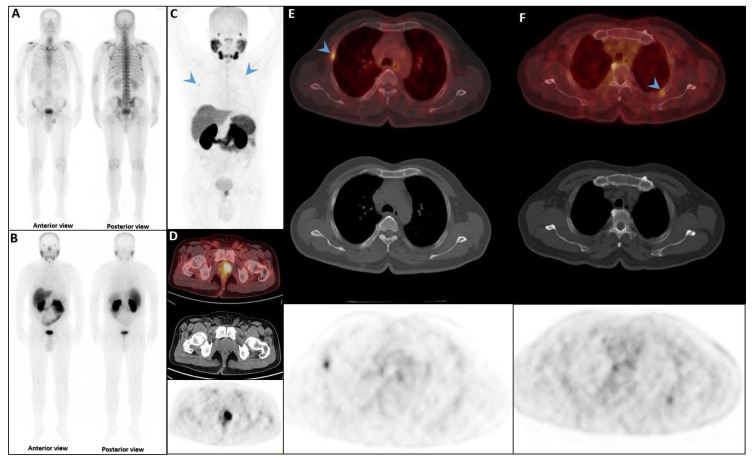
Complete discordant findings between PSMA and bone scan. 61-year-old male referred for staging that transrectal ultrasound-guided prostate biopsy showed GS 4 + 3 (12 of 12 cores involved), perineural invasion (+), and serum PSA level = 17 ng/mL. A whole-body bone scan revealed multiple increased tracer uptake in bilateral rib (A). 99mTc-PSMA SPECT/CT revealed no abnormal tracer uptake (B). Considering discordant findings in the 99mTc-PSMA and bone scan, the referring physician decided to request a 68Ga-PSMA PET/CT. The scan showed (C) multiple foci of increased activity in the left side of the prostate gland (D) as well as two foci of increased activity in bilateral ribs with no density changes on the corresponding CT images (SUVmax up to 3.84) (blue arrowhead, E,F). The rib lesions were finally considered as equivocal. Ultimately, the patient underwent RP and nadir PSA was 0.02 ng/mL.
